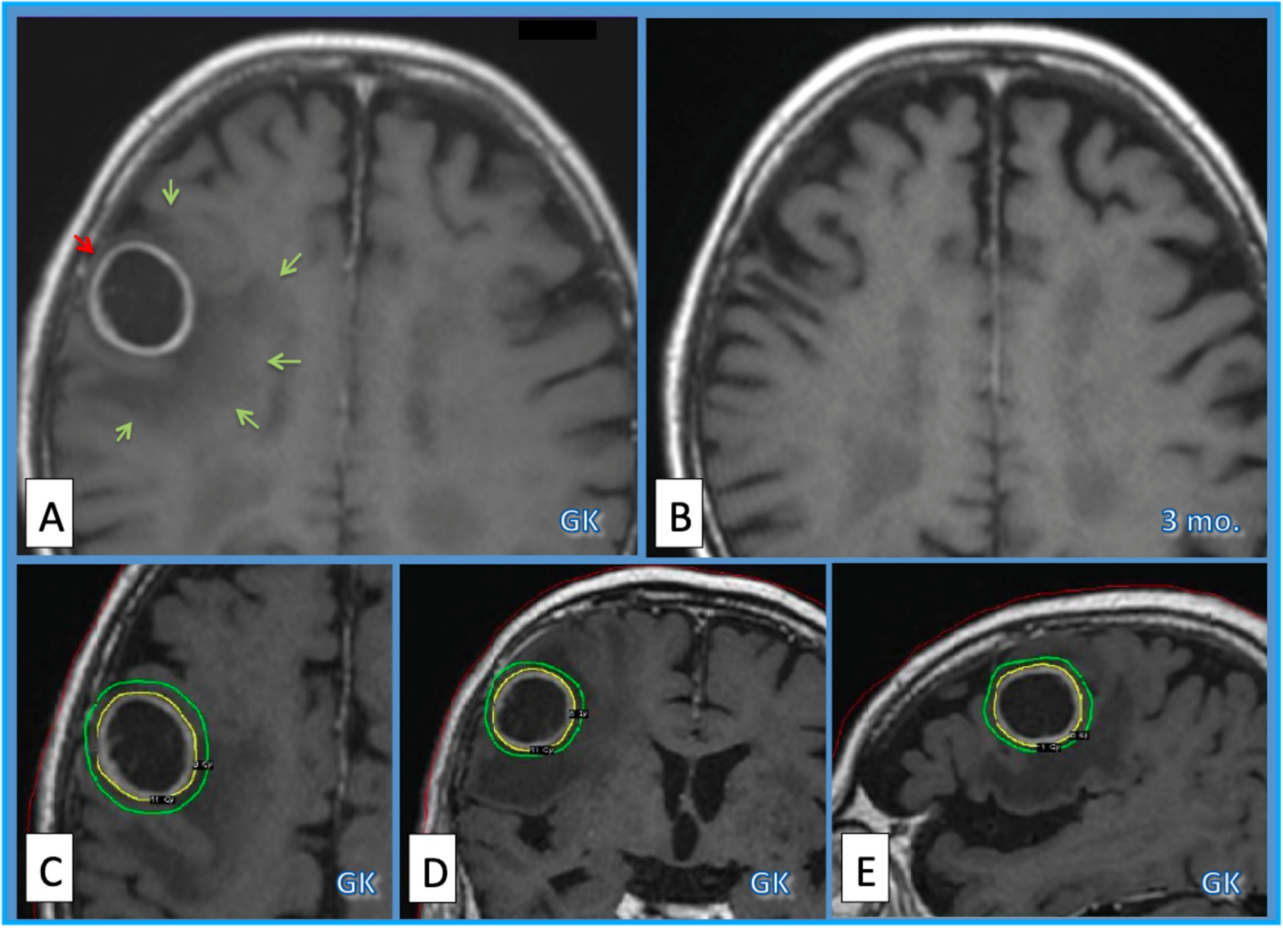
Case 10. Case 10.  Eighty-five-year-old man with bile duct carcinoma brain metastasis.  Gd-enhanced MRI before GKRS (A) showed a right frontal mass lesion (red arrow) causing perifocal edema of low-intensity. Three months after GKRS (B) the lesion disappeared and the perifocal edema (green arrows) improved completely. Dose planning of GKRS (margin dose=20 Gy/ 2fx., PIV=15.9 ml) on GammaPlan (C, axial; D; coronal; E, sagittal). The yellow line shows prescription isodose, and green line is the isodose line of 16 Gy/ 2 fx. MRI = magnetic resonance imaging, Gd = gadolinium, GKRS = Gamma Knife radiosurgery, PIV = prescription isodose volume, fx. = fraction
